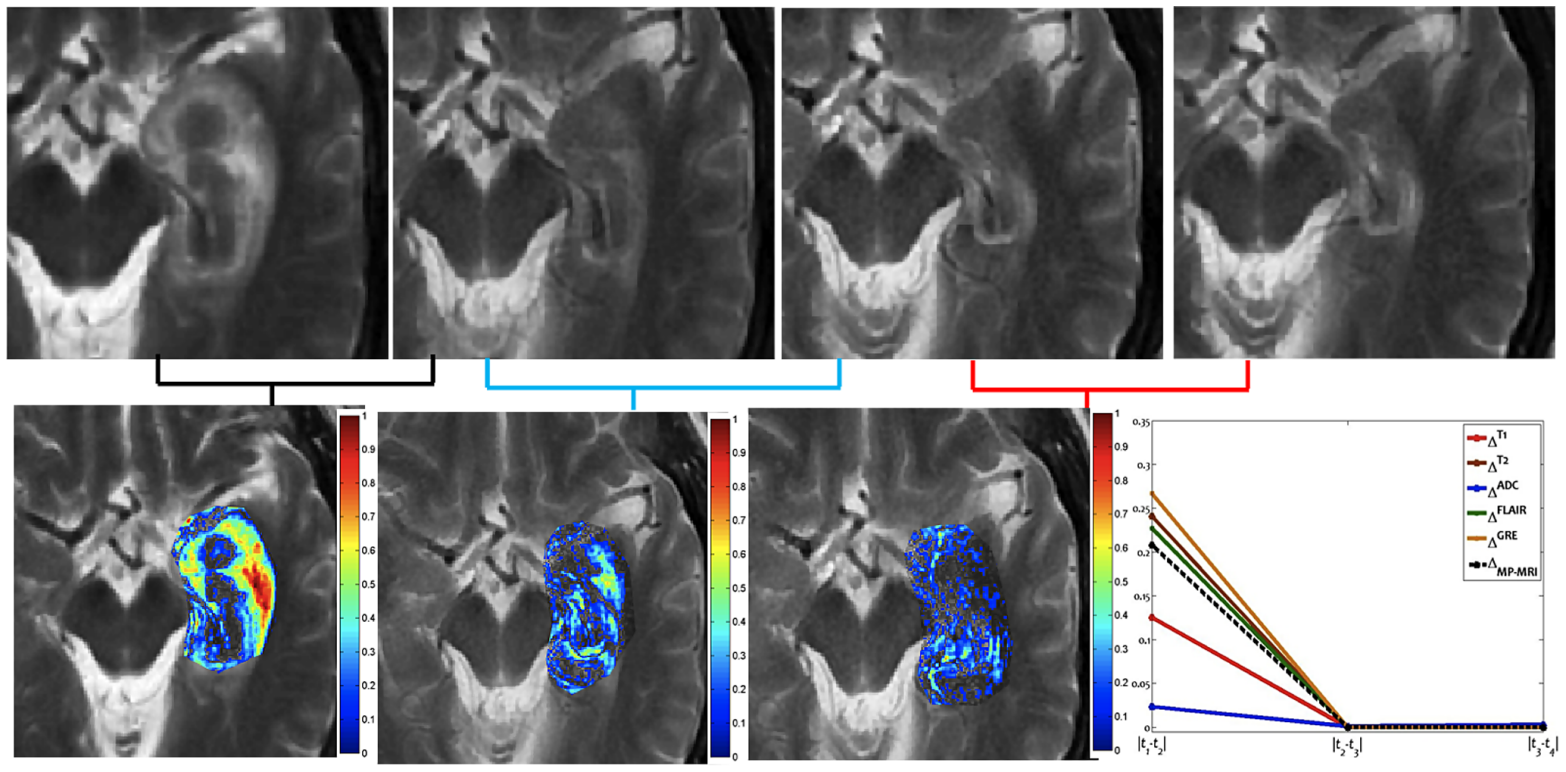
Original T2-w MRI images for (a) 24-hour, (b) 1-month, (c) 3-month, and (d) 6 month post-LITT. (e)
